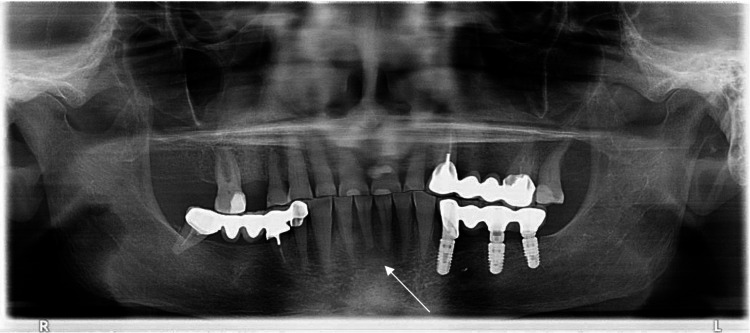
Panoramic radiograph during the emergency consultation showing no bone involvement
